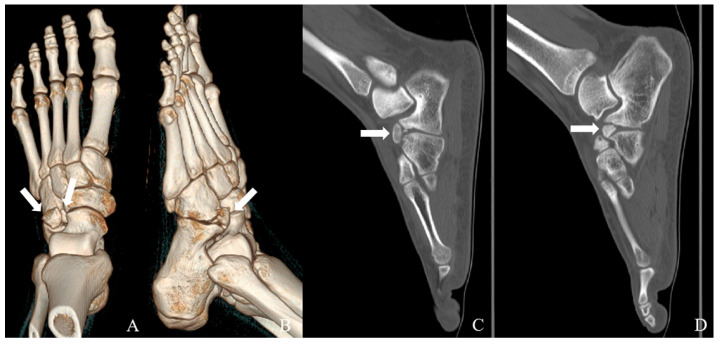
Computed tomography images of the left foot demonstrating multiple calcaneus secundarius ossicles. (A,B) Three-dimensional reconstructed images show two separate, well-corticated accessory ossicles adjacent to the anterior process of the calcaneus (arrows). (C,D) Sagittal CT images demonstrate that both ossicles have smooth cortical margins without bony continuity with the calcaneus, confirming the absence of osseous coalition.
